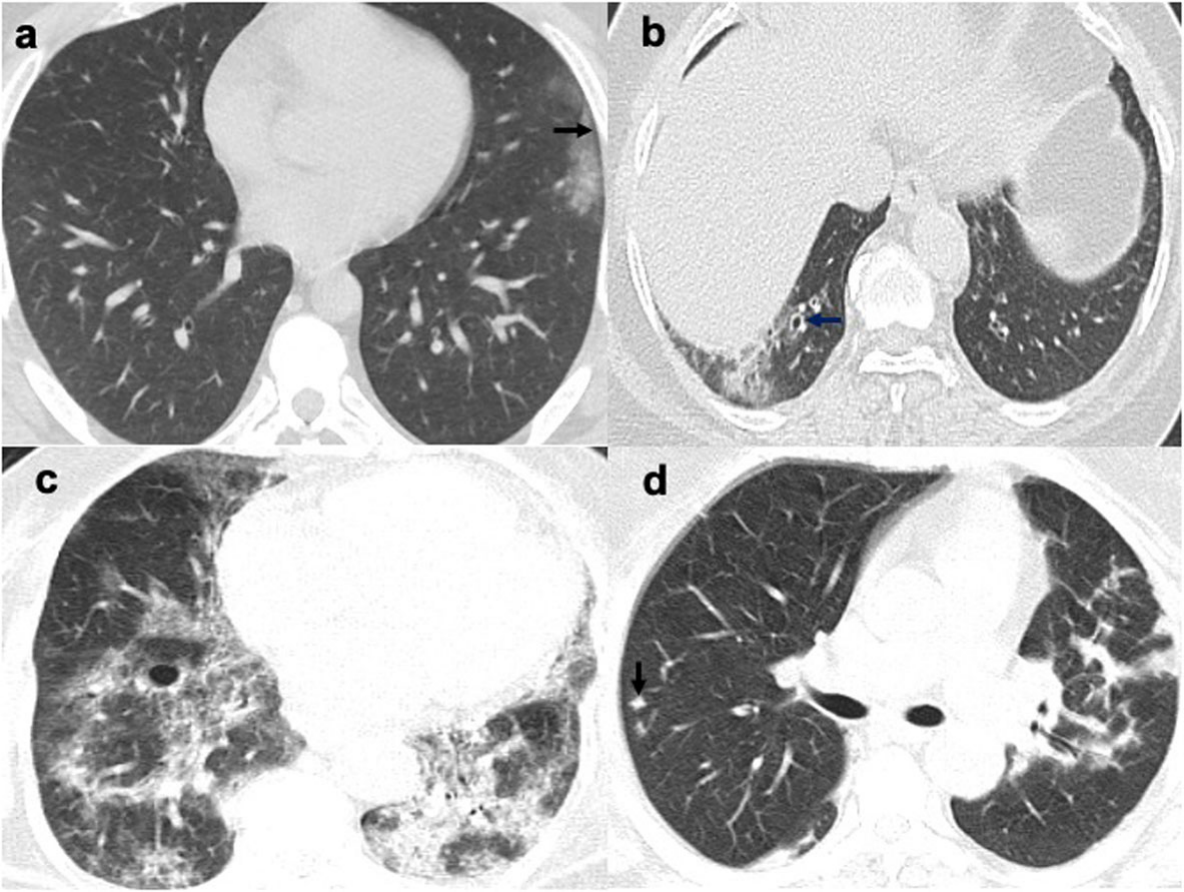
Axial chest CT lung window in cases with COVID-19 pneumonia. a The patchy ground-glass density with subpleural lucent line (arrow). b Right patchy ground-glass opacity, bronchial dilatation, and thickening (arrow). c The bilateral ground-glass densities and consolidation, with a right lower lobe cyst and a right apical segment LL angulated shape well-defined nodule (d)
